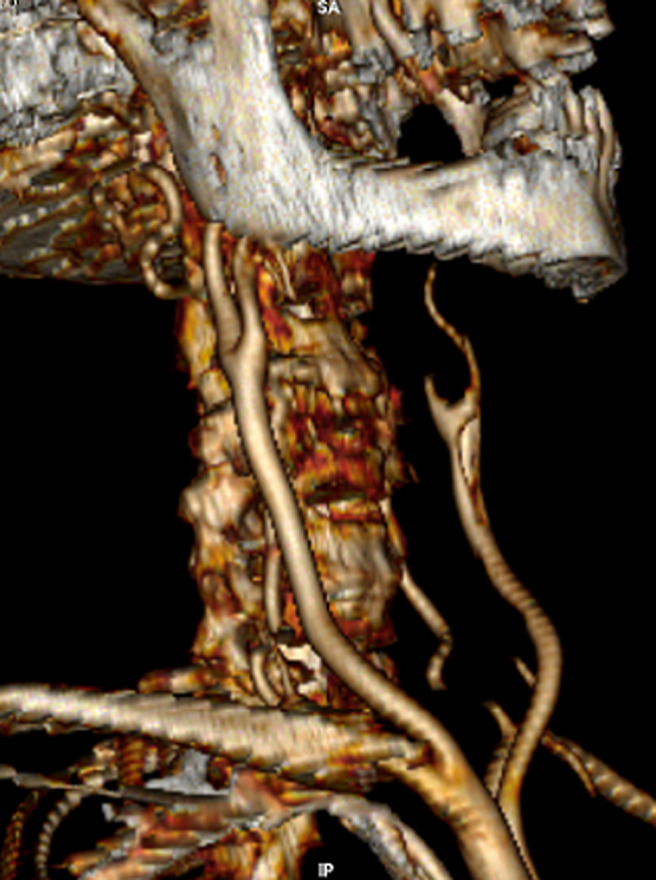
computed tomography angiography of cervical vessels shows thrombosis within the right common carotid artery extending to the internal carotid artery
